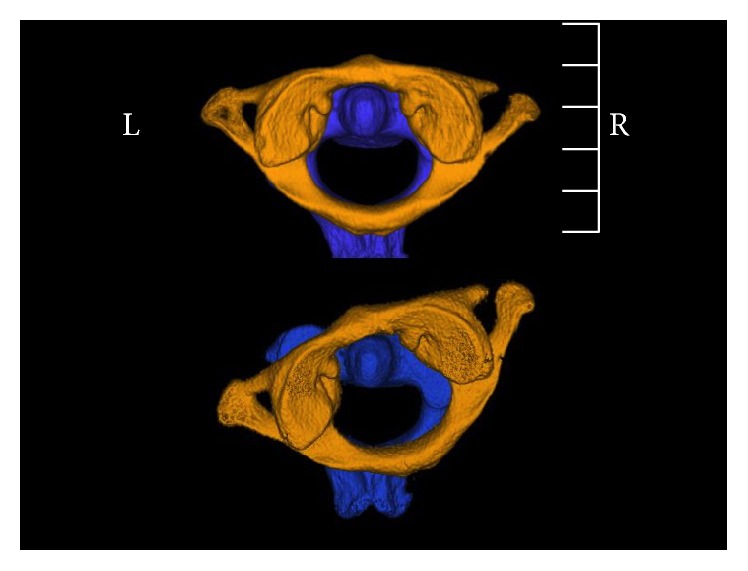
Cranial view after and before reduction in CT-VRT with segmentation of C1 and C2.
